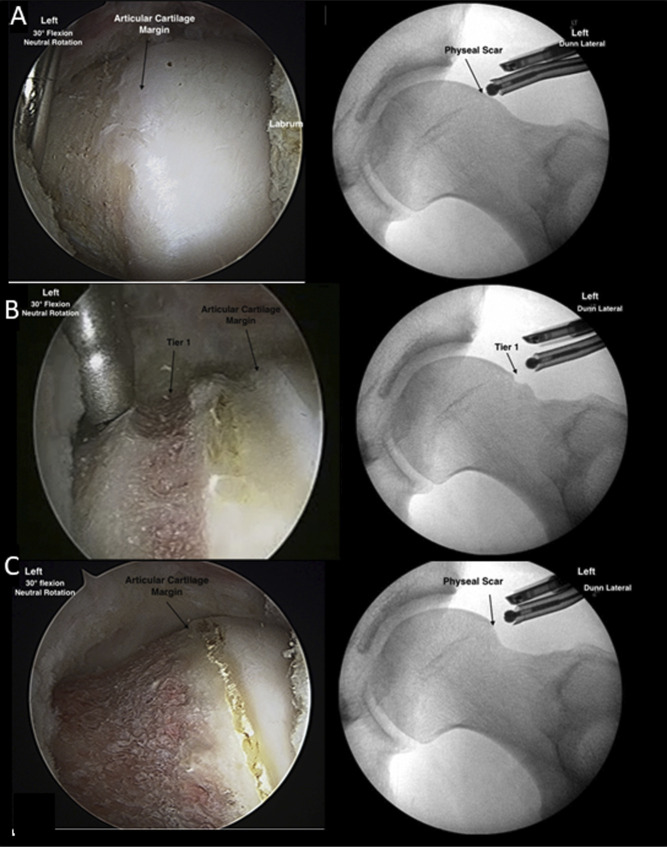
Image detailing arthroscopic and corresponding radiograph details of the two-tiered resection. A, Image demonstrating the preresection arthroscopic and fluoroscopic images in 30° of flexion and neutral rotation. B, The first tier of the resection demonstrating 1.5 burr lengths from the articular margin (C) Image demonstrating completion of the second tier of the resection and smoothing. Reproduced from Haase et al 2021 with permission from Journal of Arthroscopic Techniques.
